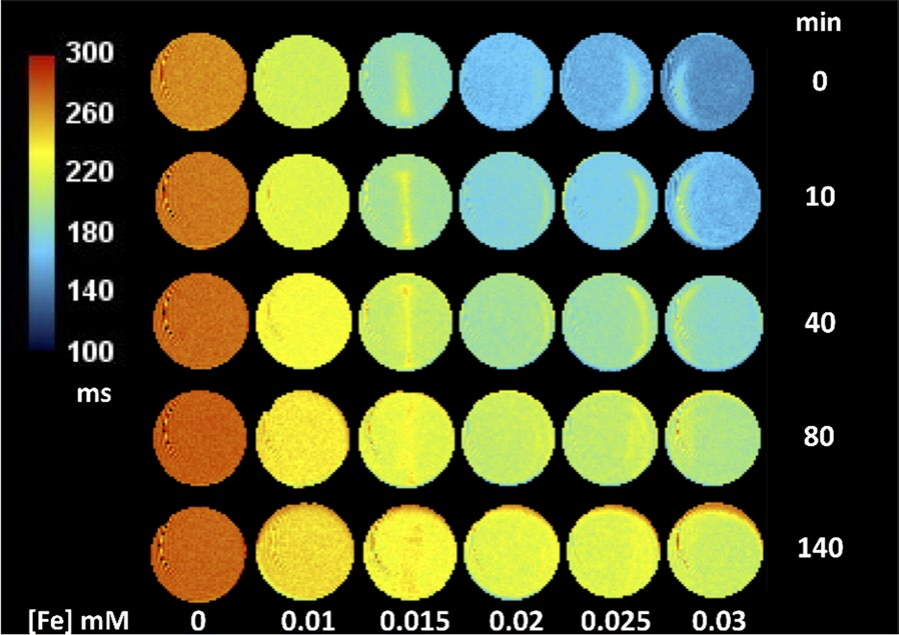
T_2_ parametric maps (2D slices showing the section) of tubes filled with APTES-NWs aqueous solutions at five different concentrations (x-axis), acquired at five selected time points (y-axis) in a 7 T MRI. The first column at the left side contains HPLC grade water and was used as a control
